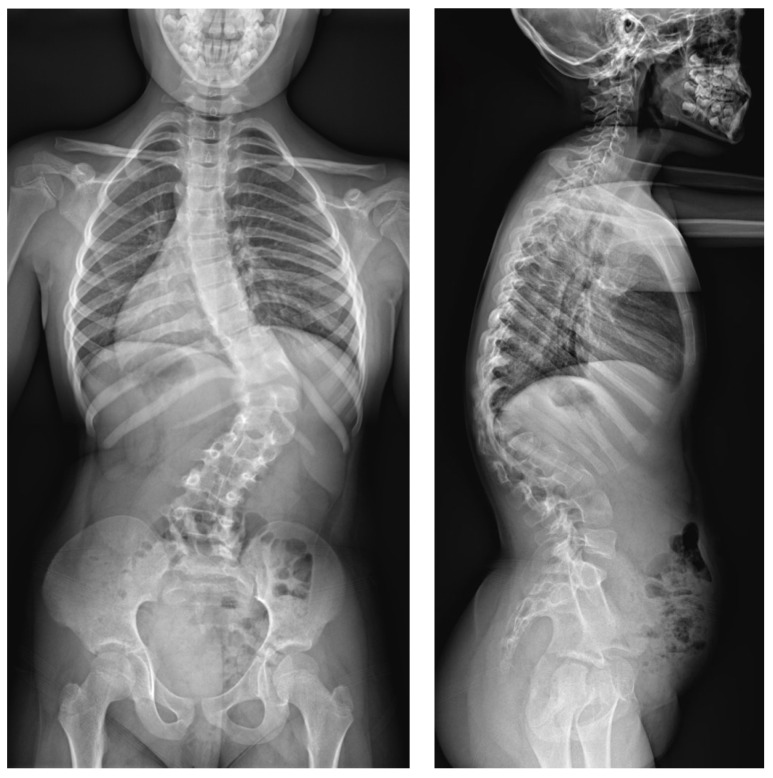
Preoperative images in a standing position: (from (left) to (right)) AP and LAT X-rays.
